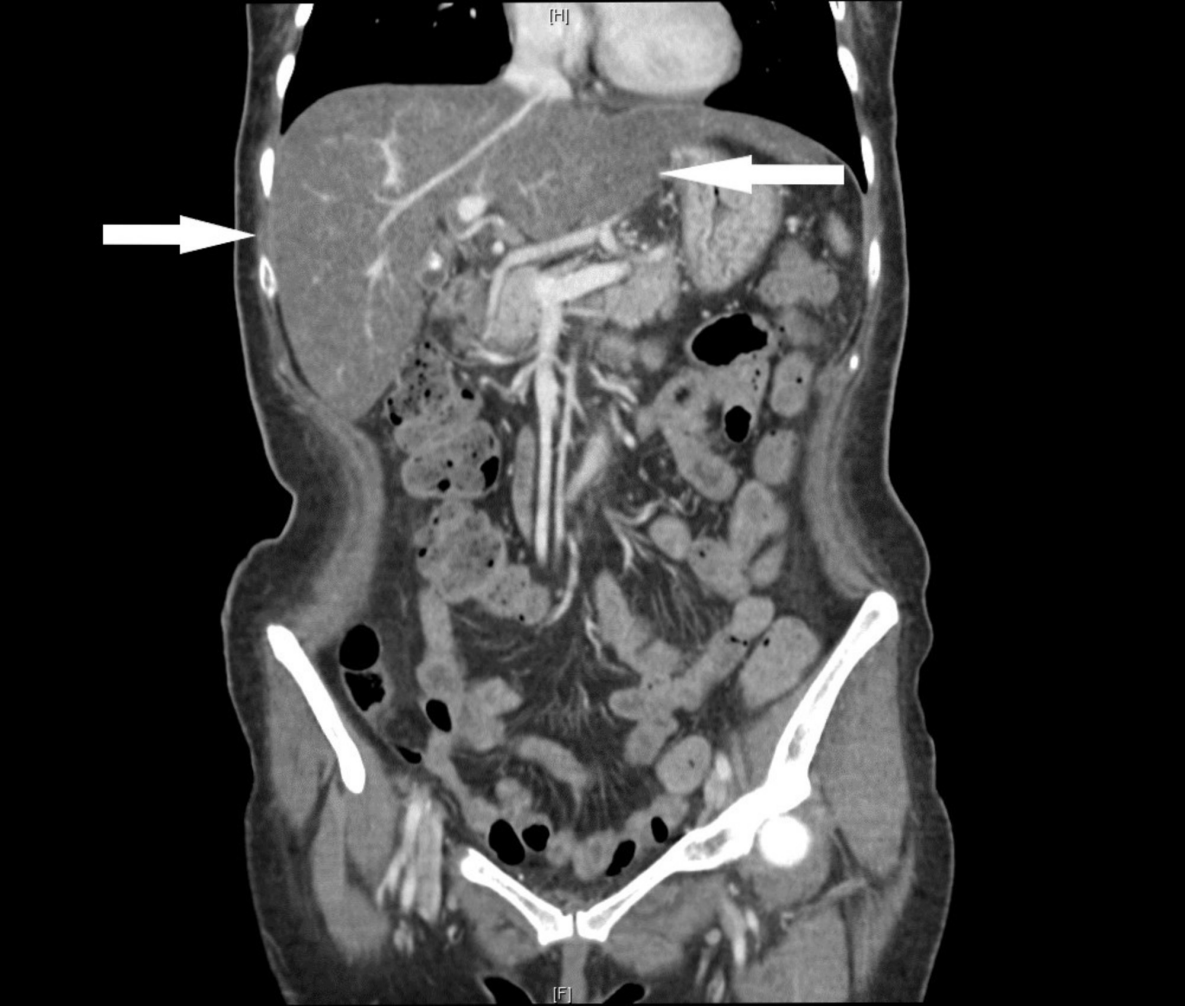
Computed tomography (CT) of the abdomen and pelvis with intravenous contrast on admission, showing hepatomegaly (marked by arrows)
